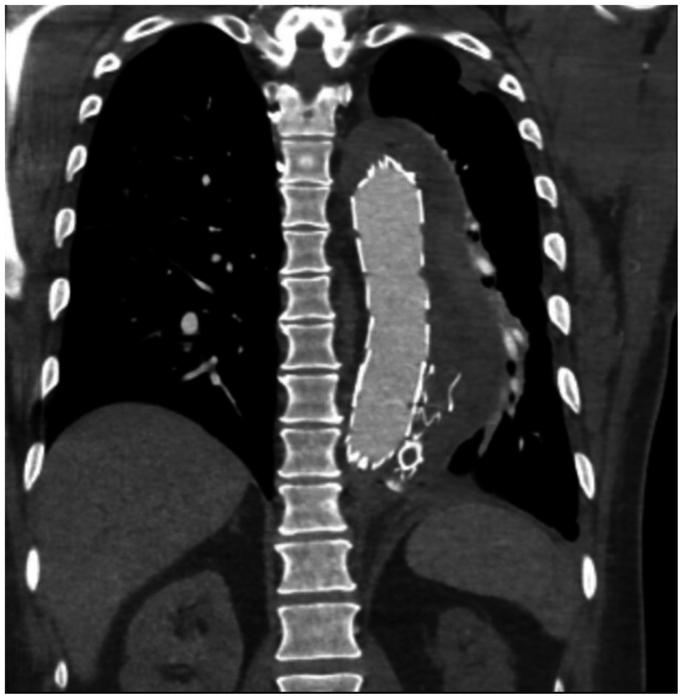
Follow-up computed tomography angiography (CTA) 7 weeks post false lumen (FL) exclusion procedure. A sagittal image demonstrating Cook converter graft and Amplatzer plugs within FL, which has completely thrombosed. The hemothorax has resolved.
